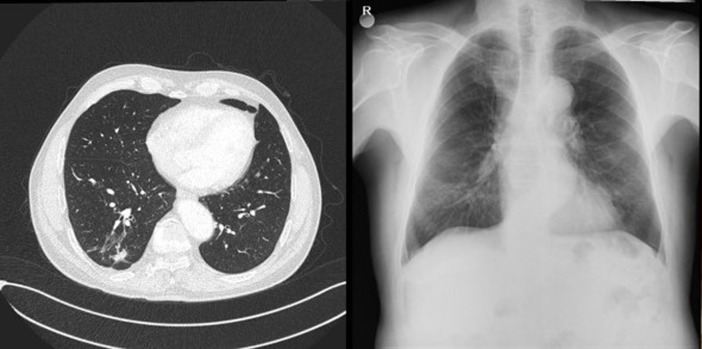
significant regression in control X-ray graphy and CT images
